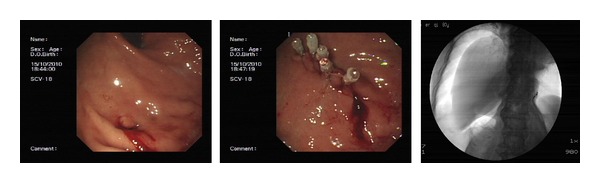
The fundus perforation was sutured by clips. The gas in peritoneal cavity was shown by X-ray.
